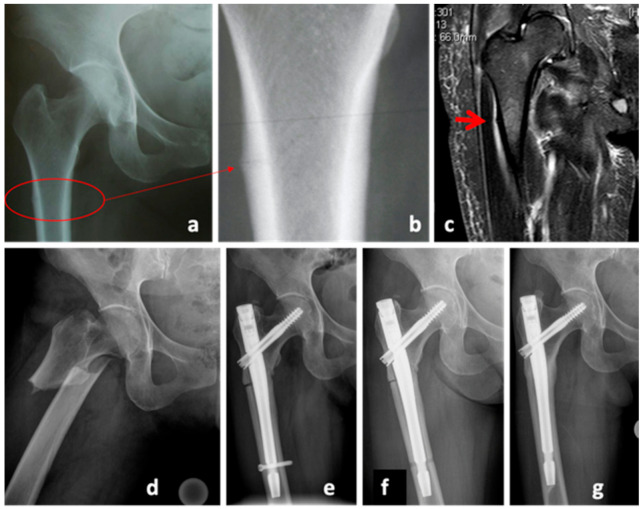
Case 1, X-Rays and MRI of the femur on the day of initial presentation (a–c) and one day later (d), showing the diagnosis of an atypical femoral fracture (AFF) under bisphosphonate therapy. X-ray of the hip, 7 months after trauma in the presence of a delayed union (e), after nail dynamization (f), and at the end of the teriparatide therapy (g), 31 months after trauma.
